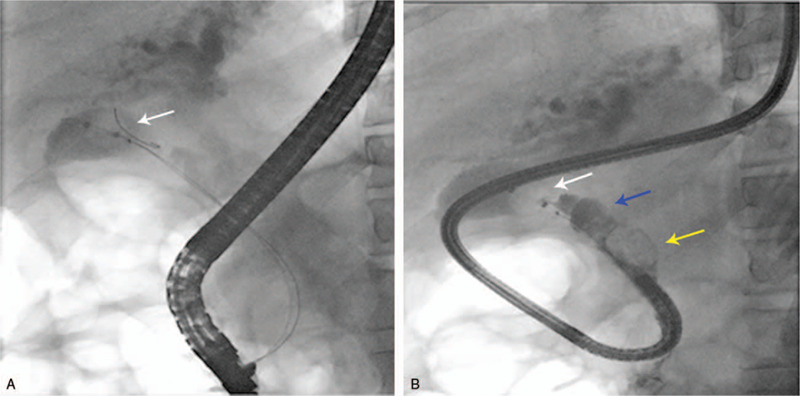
A, Radiograph showing a hybrid anchoring balloon device (white arrow) anchored in the gallbladder through the duodenoscope. B, Radiograph showing an ultraslim upper endoscope that has been passed over the anchored hybrid balloon device and advanced into the cystic duct. The impacted stone (yellow arrow), the dilated cystic duct (blue arrow), and the anchored balloon (white arrow) of the device are seen in these pictures.
